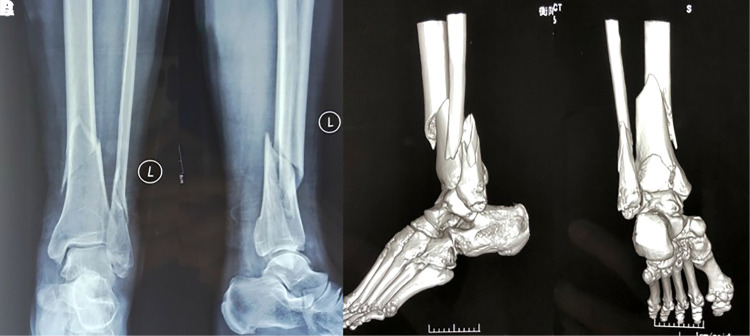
Typical case: female, 54age, fall injury, OTA fracture staging type 42-C1. (A) Preoperative X-ray orthopantomograph of distal tibial fracture; (B) Preoperative X-ray lateral radiograph of distal tibial fracture; (C,D) 3D CT of ankle showing posterior ankle fracture.
